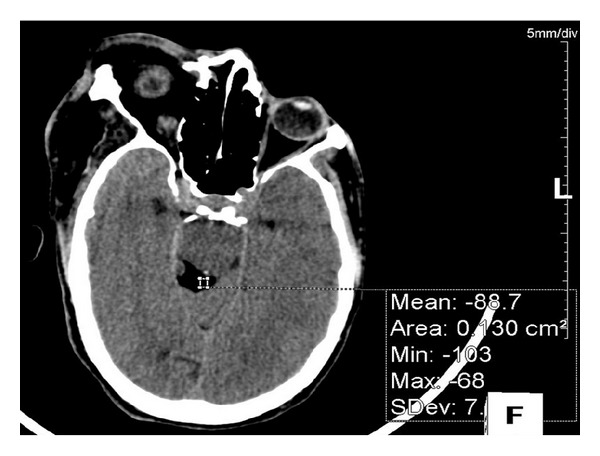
Axial CT scan showing a lipoma in the right quadrigeminal cistern.
